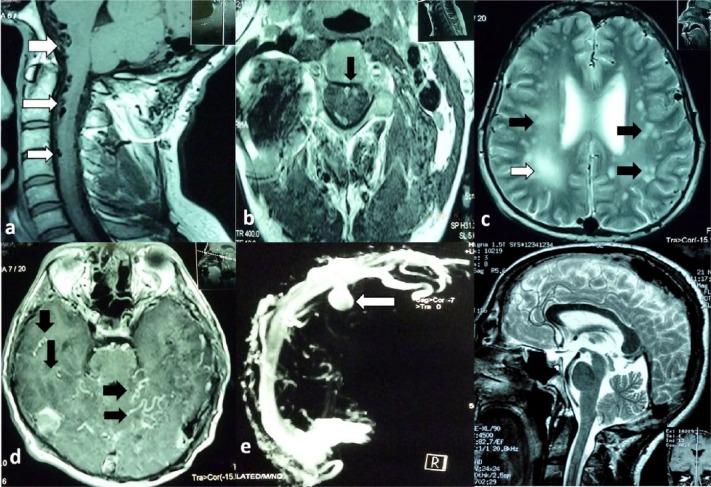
MRI of cervical spinal. (a) Sagittal T1-weighted demonstrate perimedullary venous plexus dilatation (white arrows) and venous pouch at the infratentorial level (head of white arrow). (b) Axial T1-weighted showed perimedullary dilated venous plexus. MRI of brain. (c) Axial T2-weighted reveal multiple foci of leukoaraiosis (black arrows), edema of white matter (white arrows), and venous ischemia (head of white arrow). (d) Axial with Gd showed vascular enhancement (black arrows). (e) MRI venography demonstrate presence of venous pouch at the supratentorial level (white arrows). (f) MRI brain without changes of white matter (before evolution of dural fistula).
